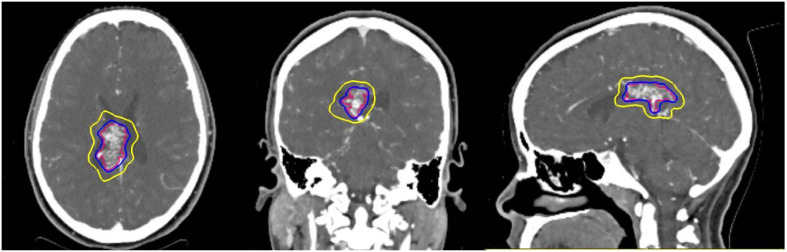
Representative stereotactic radiosurgery case with planned treatment volume (red) isodose (blue) and 50% isodose (yellow) can be seen in three planes on pretreatment planning CTA.
